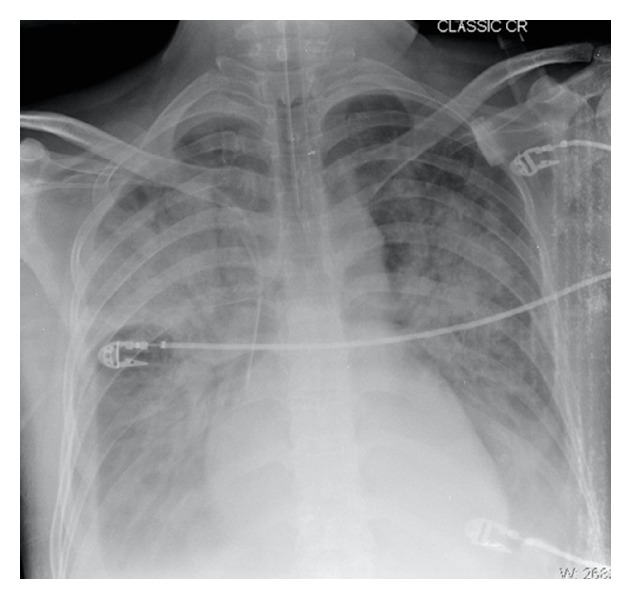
Anteroposterior chest radiography, which shows diffuse pulmonary congestion with bilateral pleural effusion.
